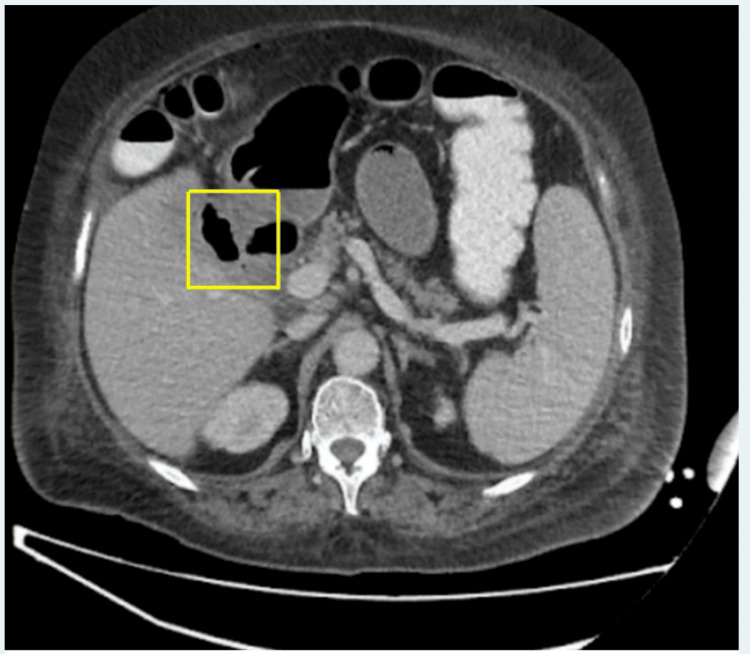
Axial CT image of the abdomen highlighting the cholecystoduodenal fistula, indicated by a yellow rectangular box Air is visible within the thickened gallbladder.
